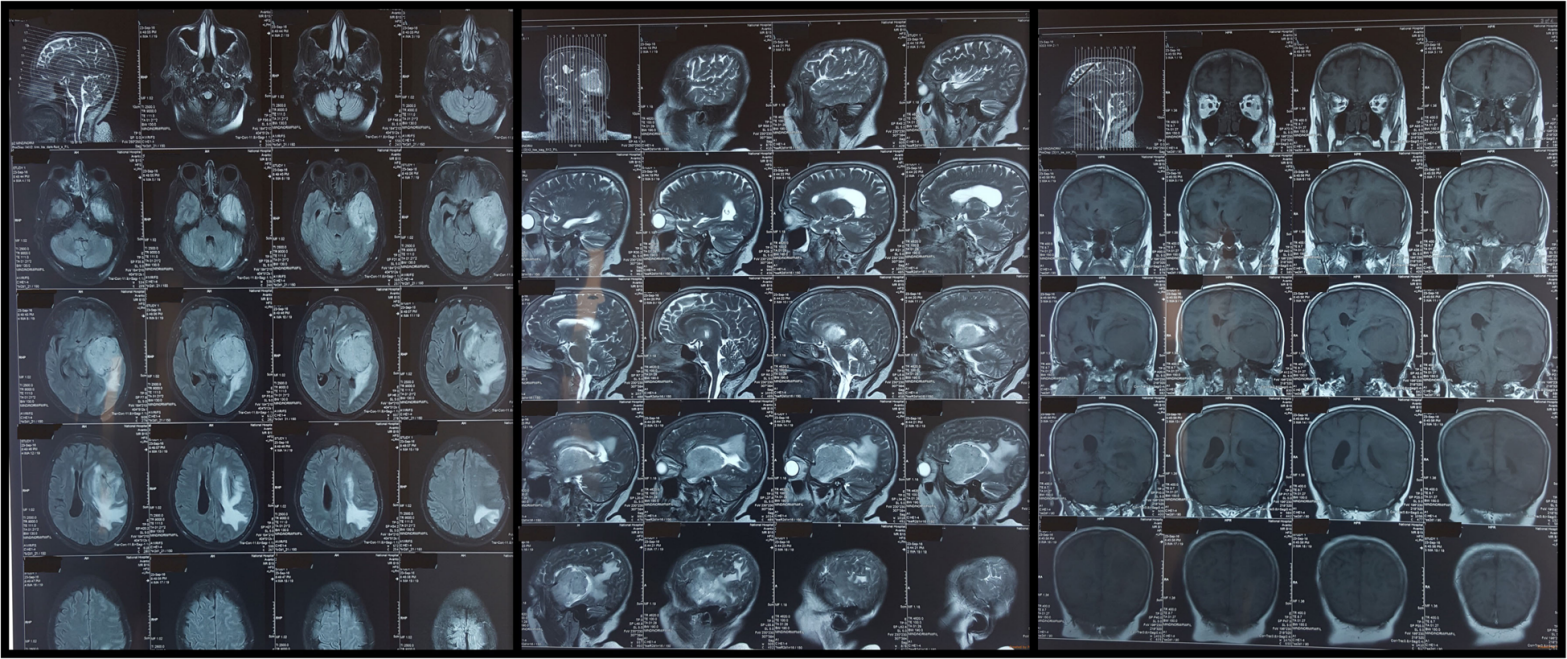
MRI images of the large left sided frontoparietal sphenoidal ridge meningioma with mass effect (Axial, sagittal and coronal view)
